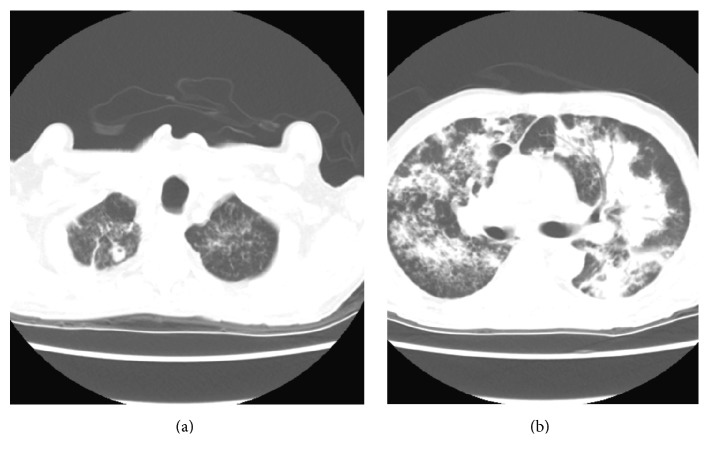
CT chest with IV contrast taken on day 13. (a) shows right upper lobe cavitary lesion. (b) shows multilobar consolidation.
